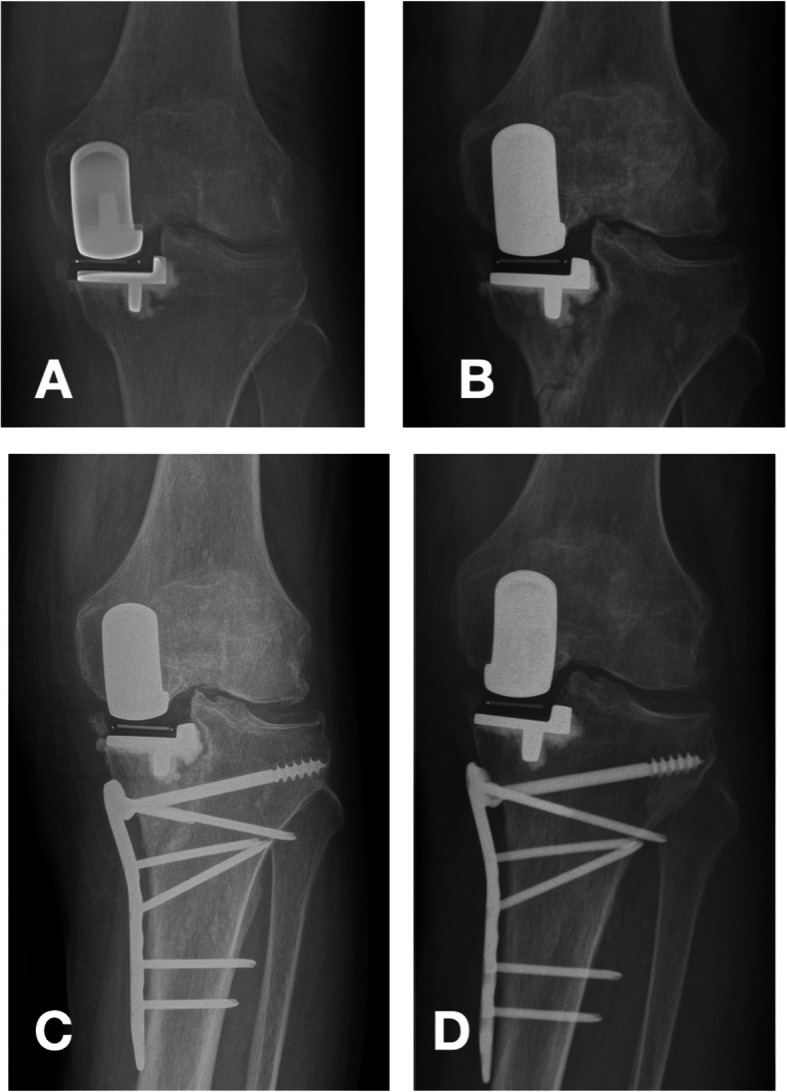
shows an 81-year-old woman fell down 2 months after surgery and later performed open reduction of tibial fracture with internal fixation. a is the X-rays of the patient 2 weeks after surgery. b is the X-rays of the patient 2 months after surgery. c is the X-ray of the patient 3 days after plate fixation. d is the X-ray of the patient 8 months after plate fixation
